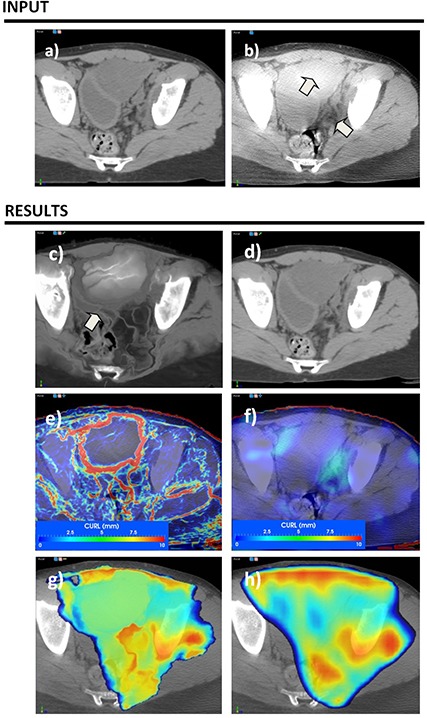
Evaluation of deformable registration as applied in adaptive radiotherapy. The CT dataset (a) was warped to the CBCT dataset (b) using a monomodality diffeomorphic demons algorithm (c), (left column in results section) and a multimodality B‐Spline algorithm (d), (right column in results section). In (c), HU calibrations and various artifacts present in the CBCT dataset violated the monomodality assumption of the first algorithm, leading to unrealistic warping. In (d), the solution provided by algorithm 2 interpolates artifacts but is not able to model small local organ changes. The vortex map easily catches these characteristics of the displacement fields (e) and (f). When warping the dose distribution from the planning to the CBCT dataset, the distribution warped by algorithm 1 looks unnatural and unrealistic (g), while the dose distribution warped by algorithm 2 looks natural (h).
